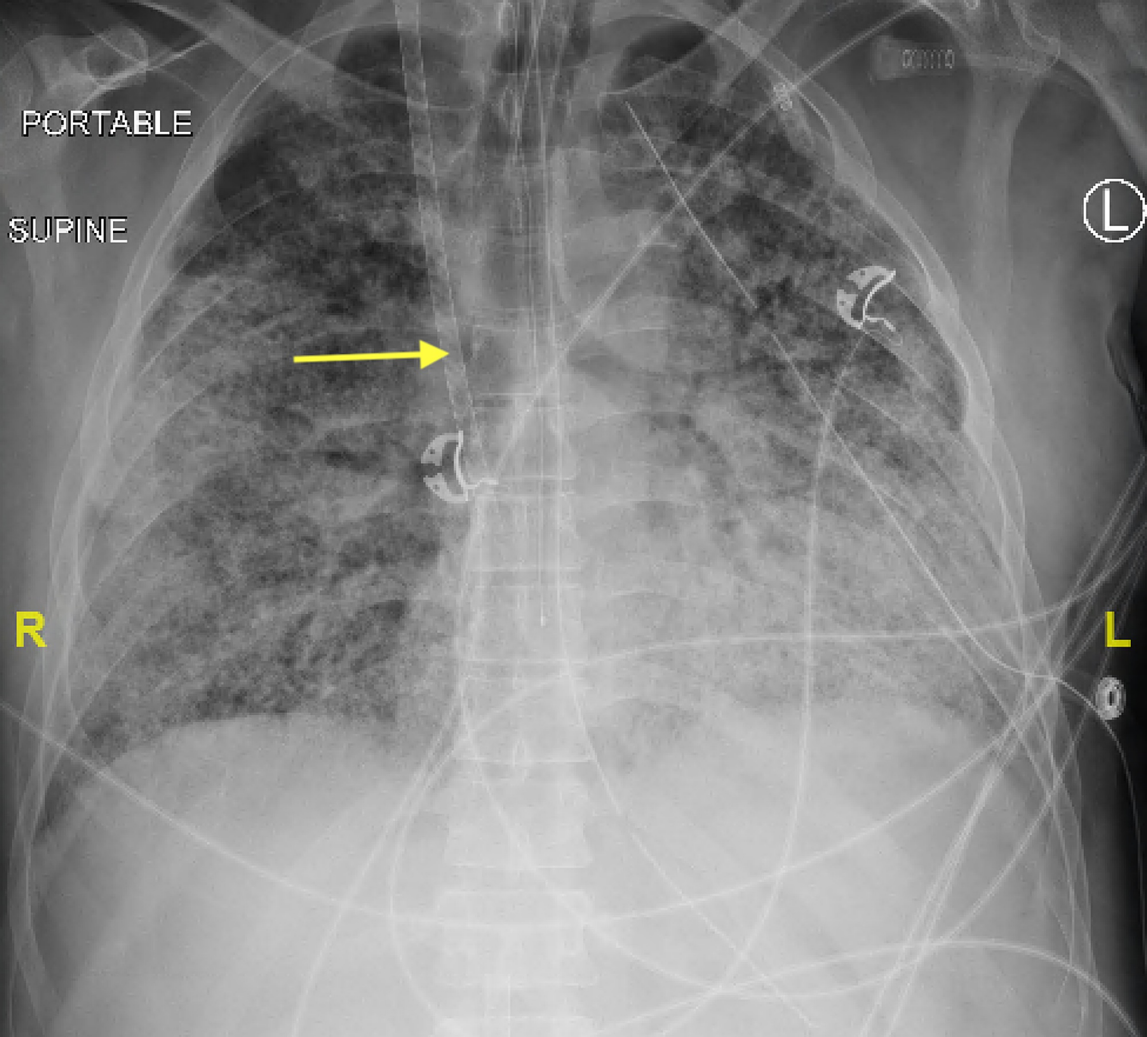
Portable supine anteroposterior radiograph of the chest demonstrate presence of right internal jugular catheter (yellow arrow) terminating in the right atrium. No evidence of pneumothorax. Cardiomediastinal contours are difficult to evaluate due to overlying opacities.
